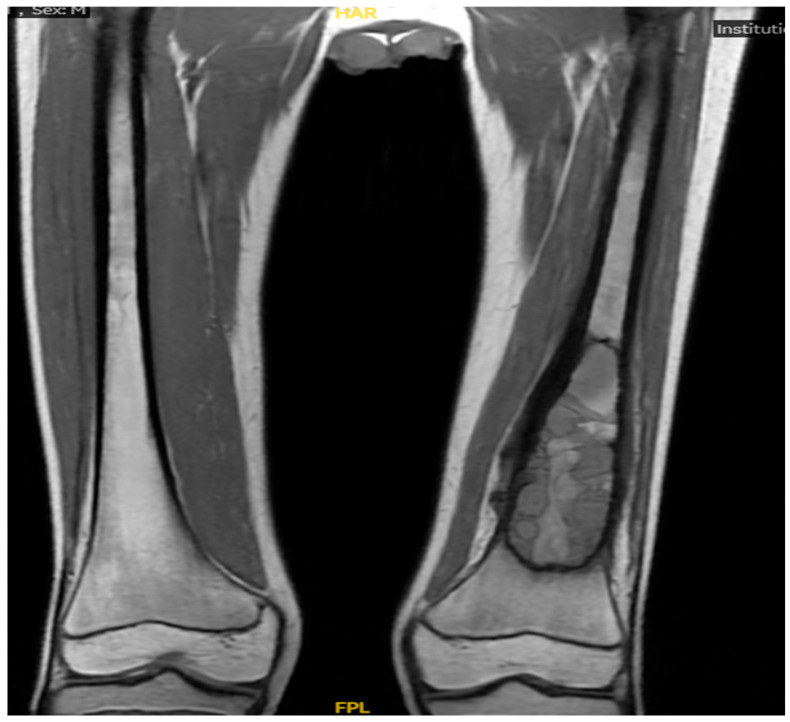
Magnetic resonance imaging of the left femur demonstrating an aggressive intramedullary lesion with cortical breach and soft tissue extension.
